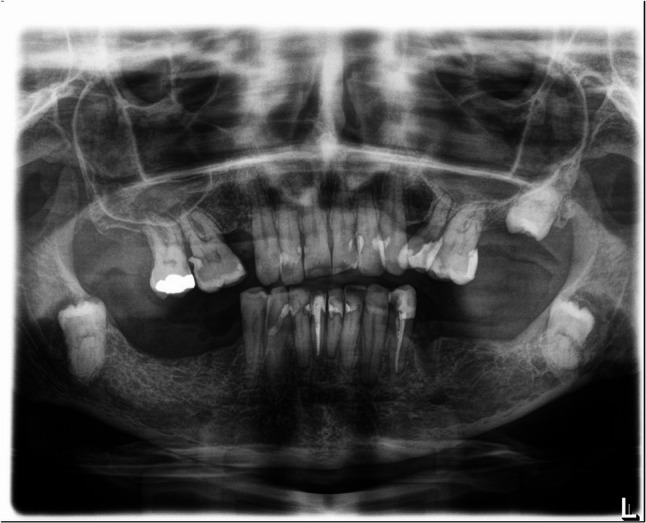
Panoramic radiograph of the same patient 7 years after radiotherapy, multiple new restorations in upper front and premolar regions, endodontic treatment of teeth 35 and 41 and extractions of teeth 14, 27, 37, 45 and 47 have been performed, significant bone loss is visible in the molar regions of the mandible compared to the earlier radiographs
